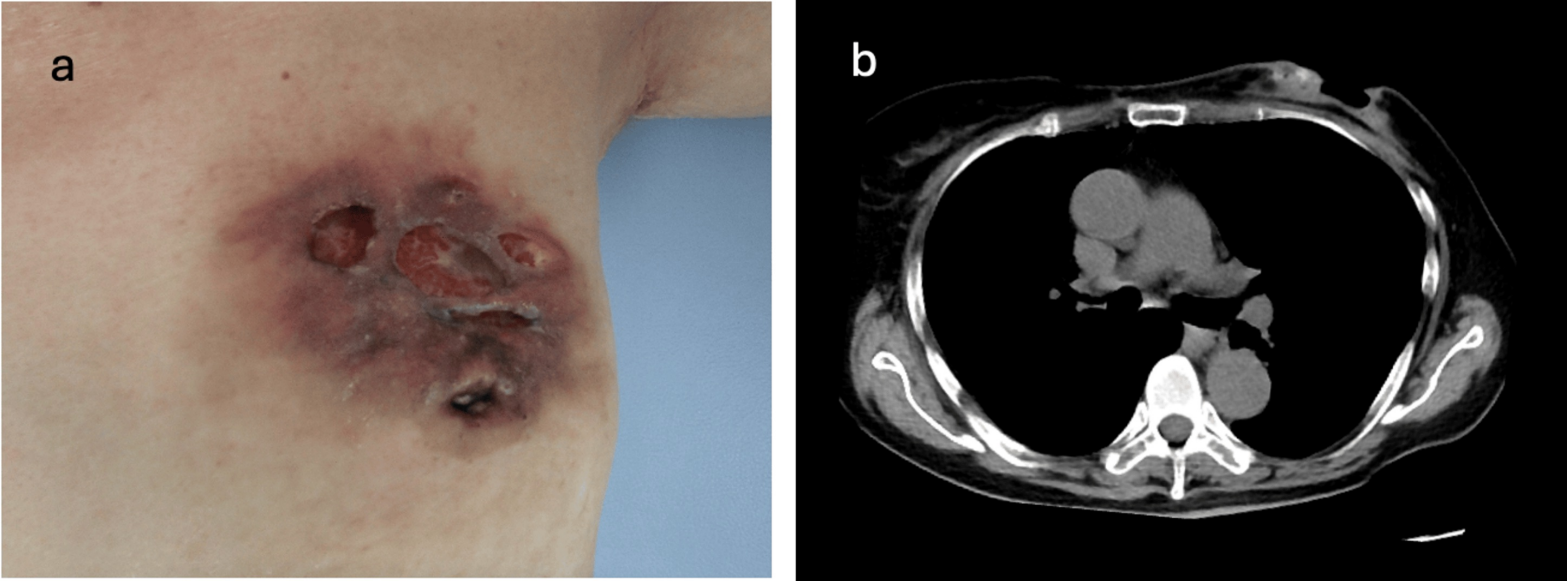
(a) Patient photograph taken 37 days after cardiopulmonary arrest (CPA). (b) CT images showing tumor regression to 40 mm.
